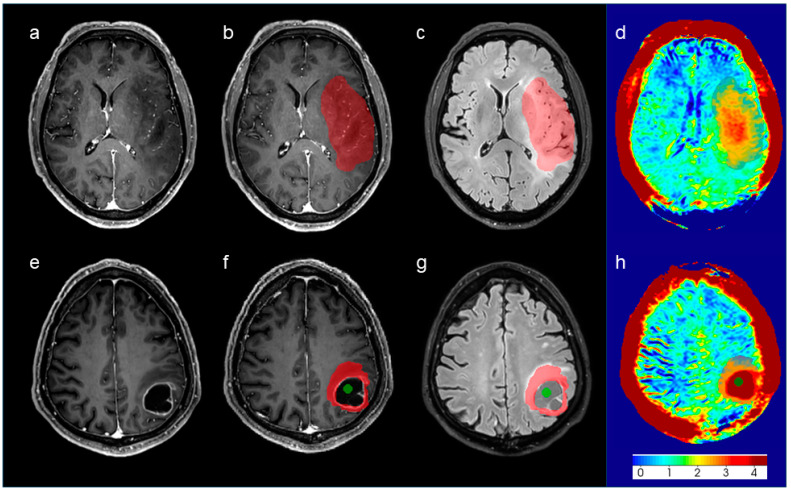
Diffuse astrocytoma grade 2, IDH-mutant, (a–d) and glioblastoma, IDH-wildtype (e–h), are shown. Images in the first two columns (a,b,e,f) and in the third column (c,g) represent post-gadolinium volumetric T1 sequences and FLAIR volumetric images, respectively. Images in the fourth column (d,h) show the corresponding color-coded APT signal. Both patients presented with space-occupying lesions within the left cerebral hemisphere, the second showing necrotic components. Red ROI represents the solid component (“lesion ROI”), while green ROI represents the necrotic component (“necrosis ROI”).
